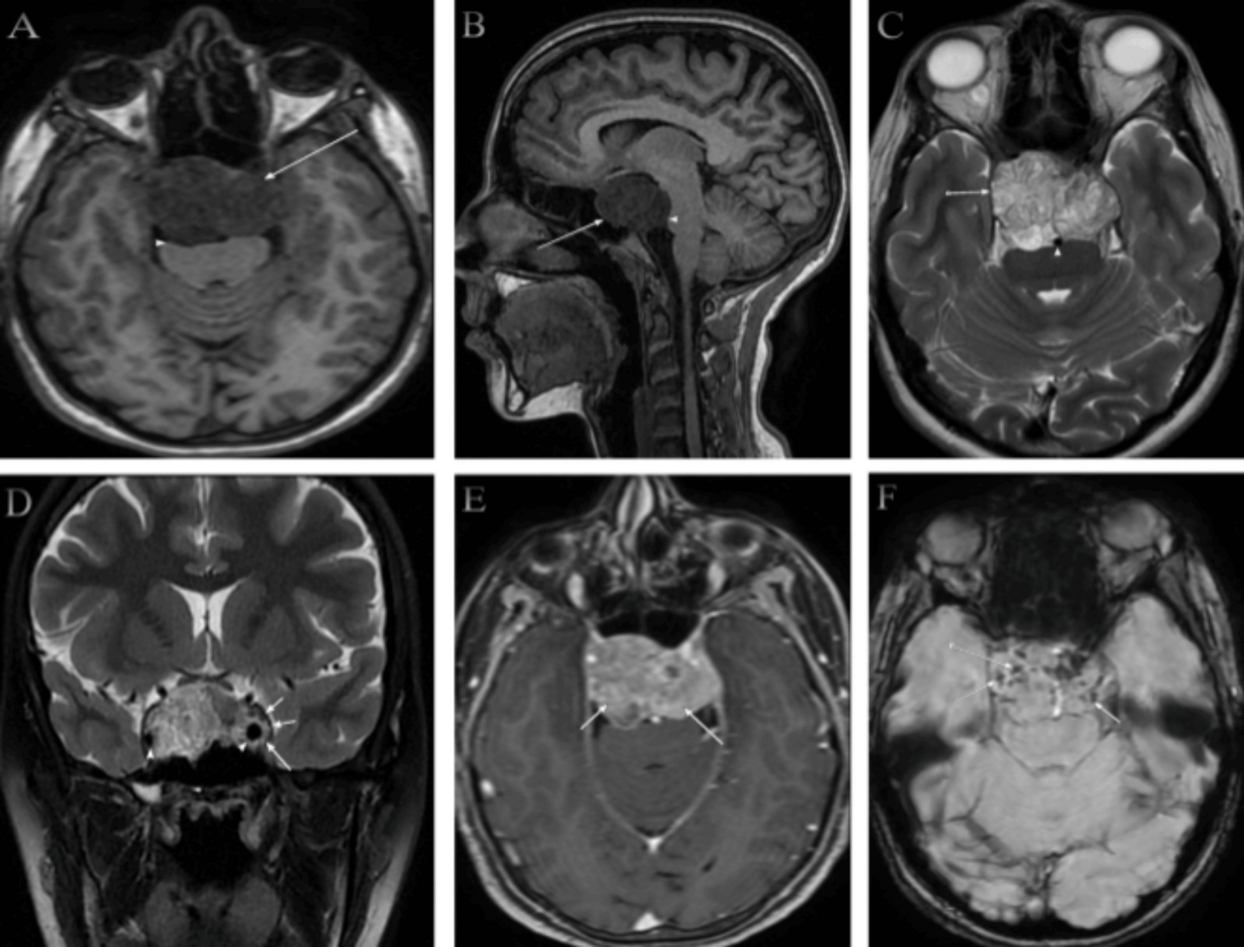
Preoperative brain magnetic resonance imaging (MRI) T1-weighted images on axial (A) demonstrate predominantly intermediate signal intensity (long arrow) with significant mass effect on the brain stem (arrowhead). T1-weighted images on sagittal (B) also demonstrate predominantly intermediate signal intensity (long arrow) with significant mass effect on the brain stem (arrowhead). T2-weighted images on axial (C) demonstrate heterogeneous hyperintensity (long arrow) with partial encasement of the basilar artery without vascular narrowing (arrowhead). T2-weighted images on the coronal plane (D) show bilateral invasion of the cavernous sinuses, more pronounced on the left side (long arrow), with encasement of the internal carotid arteries bilaterally (arrowhead), without vascular narrowing. Contrast-enhanced fat-suppressed T1-weighted images on the axial plane (E) show moderate heterogeneous enhancement. Susceptibility-weighted imaging (SWI) on the axial plane (F) shows foci of susceptibility effect involving the periphery of the mass, likely representing calcification
